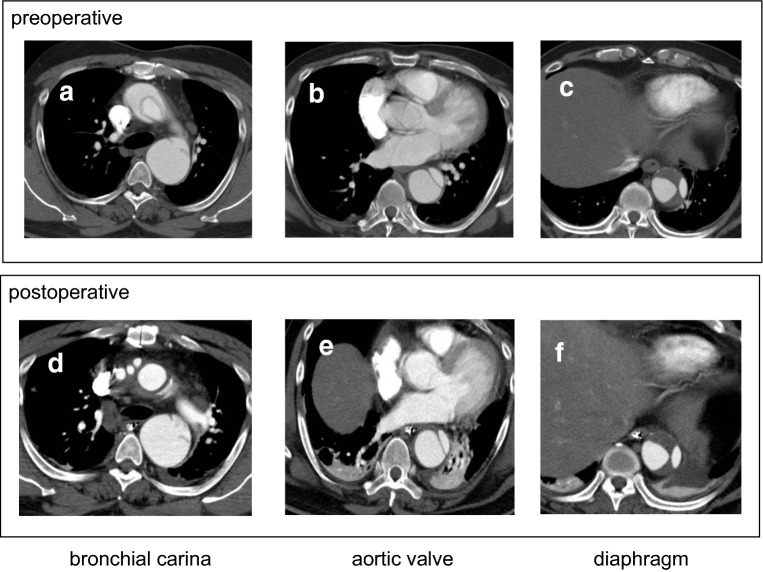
CT scan of a patient treated with TAR without FET before 2015. Preoperative image (a–c), and postoperative image before discharge from the hospital (d–f) at the level of bronchial carina (a, d), aortic valve (b, e), and diaphragm (c, f), respectively. Note that, although the elephant trunk was inserted in the descending aorta, the false lumen is patent and the true lumen expansion is not observed at each level of downstream aorta
